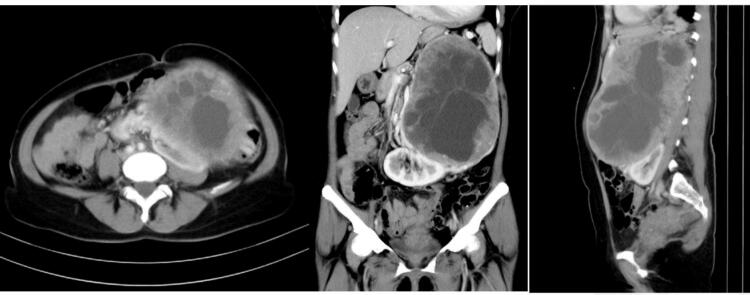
CT scan of the abdomen shows a mass at the upper region of the adrenal.
